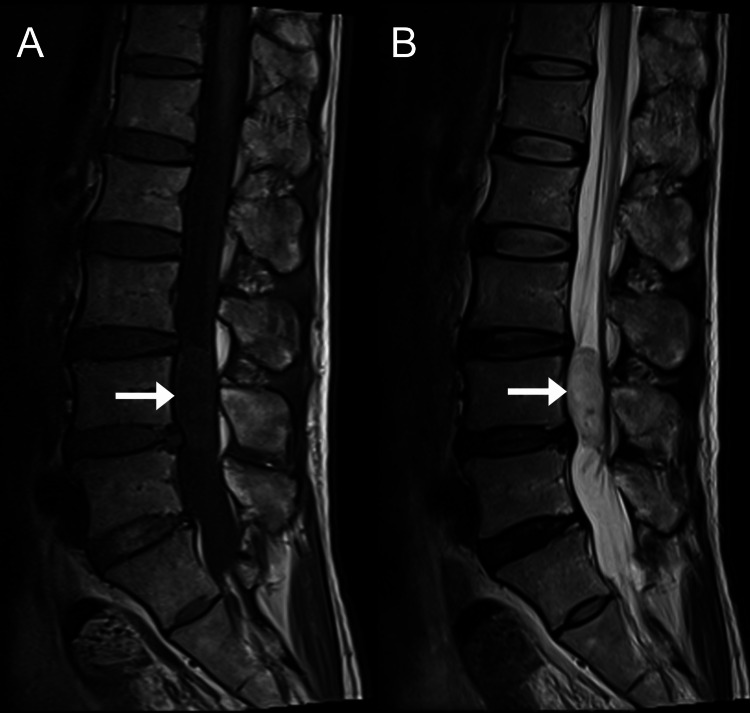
Lumbar spine magnetic resonance imaging, sagittal views. An intradural extramedullary mass is evident occupying the entire spinal canal at L4 level (arrows), measuring 1.98 cm × 1.32 cm × 3.73 cm, which is isointense on T1-weighted sequence (A), and slightly hyperintense on the T2-weighted sequence (B). Compression of the cauda equina is also evident.
